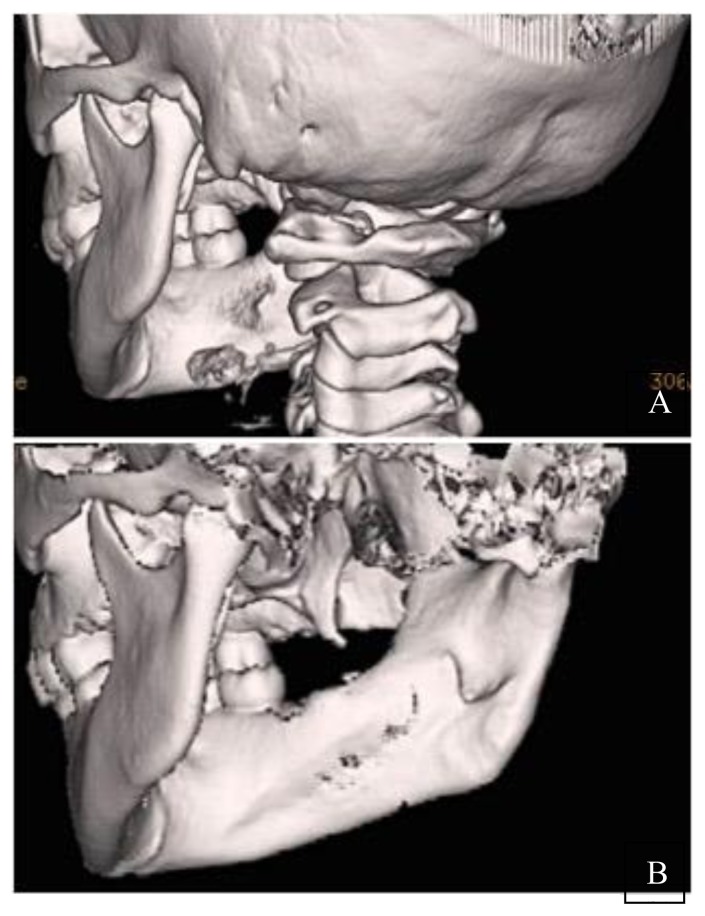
3-dimensional CT of mandible before (a) and after (b) 3 courses of pamidronate therapy. a) Osteolytic lesions in the ascending ramus, neck and condylar head of right mandible. b) Bone remodeling with thick periosteal reaction.
